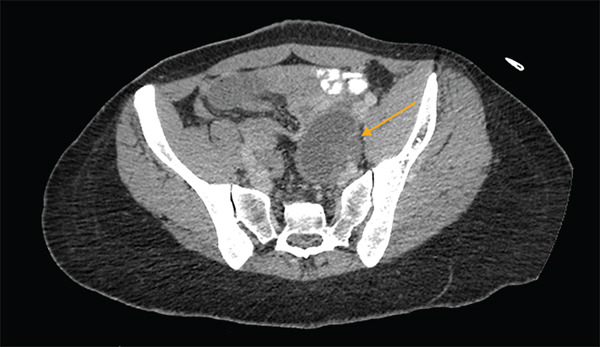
CT scan of abdomen/pelvis with IV contrast performed on admission to the emergency department on HOD 1.
